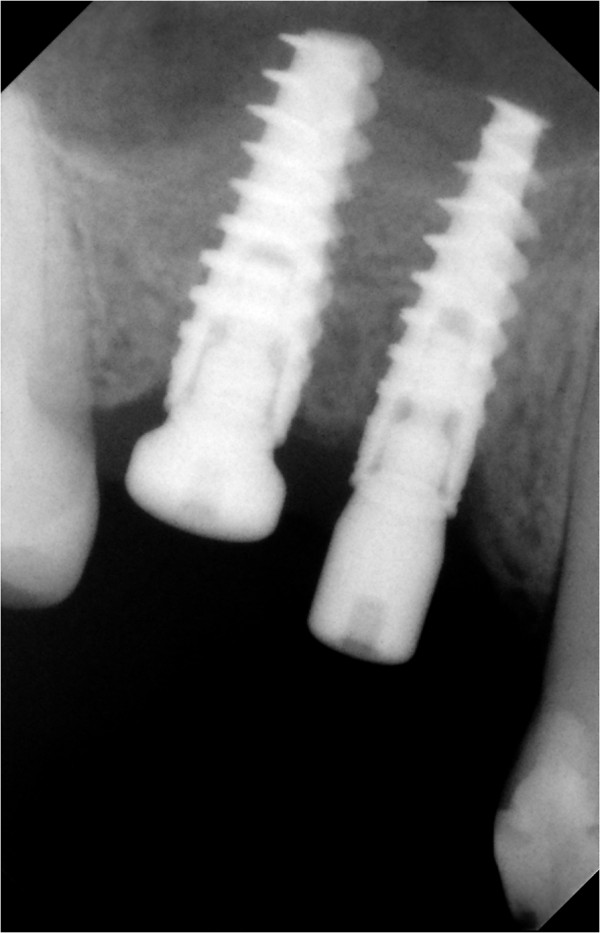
Radiographic postoperative control at six months.
